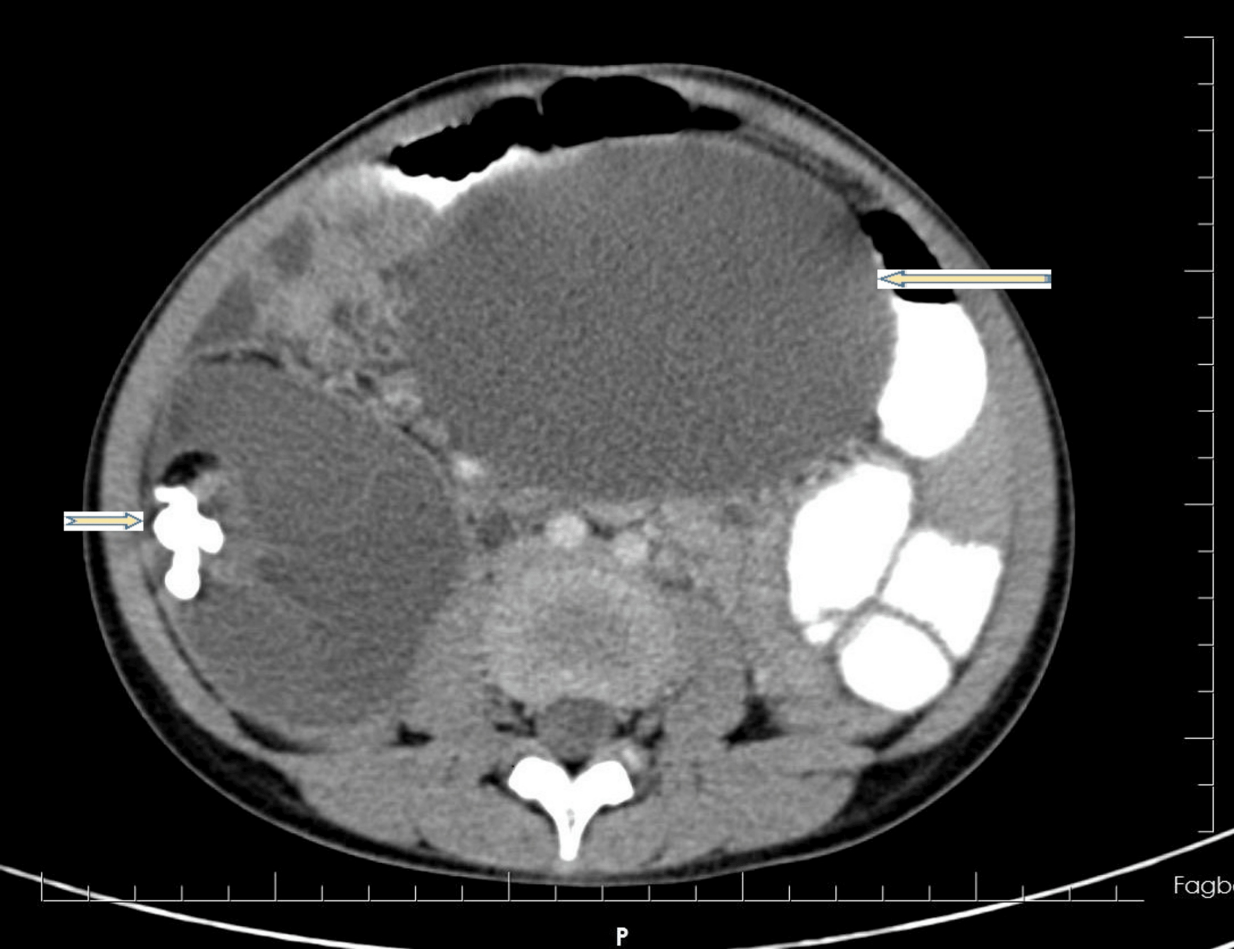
Axial contrast-enhanced CT scan of the abdomen. The image shows bilateral giant ovarian masses occupying the pelvic and lower abdominal cavities. The right-sided mass contains areas of fat and calcification (arrow), characteristic of a mature cystic teratoma. The left adnexal mass demonstrates heterogeneous density with surrounding fluid and mass effect (arrow), suggestive of ovarian torsion. The enlarged masses exert significant compressive effect on adjacent pelvic organs, correlating with the patient’s presentation of acute urinary retention.
